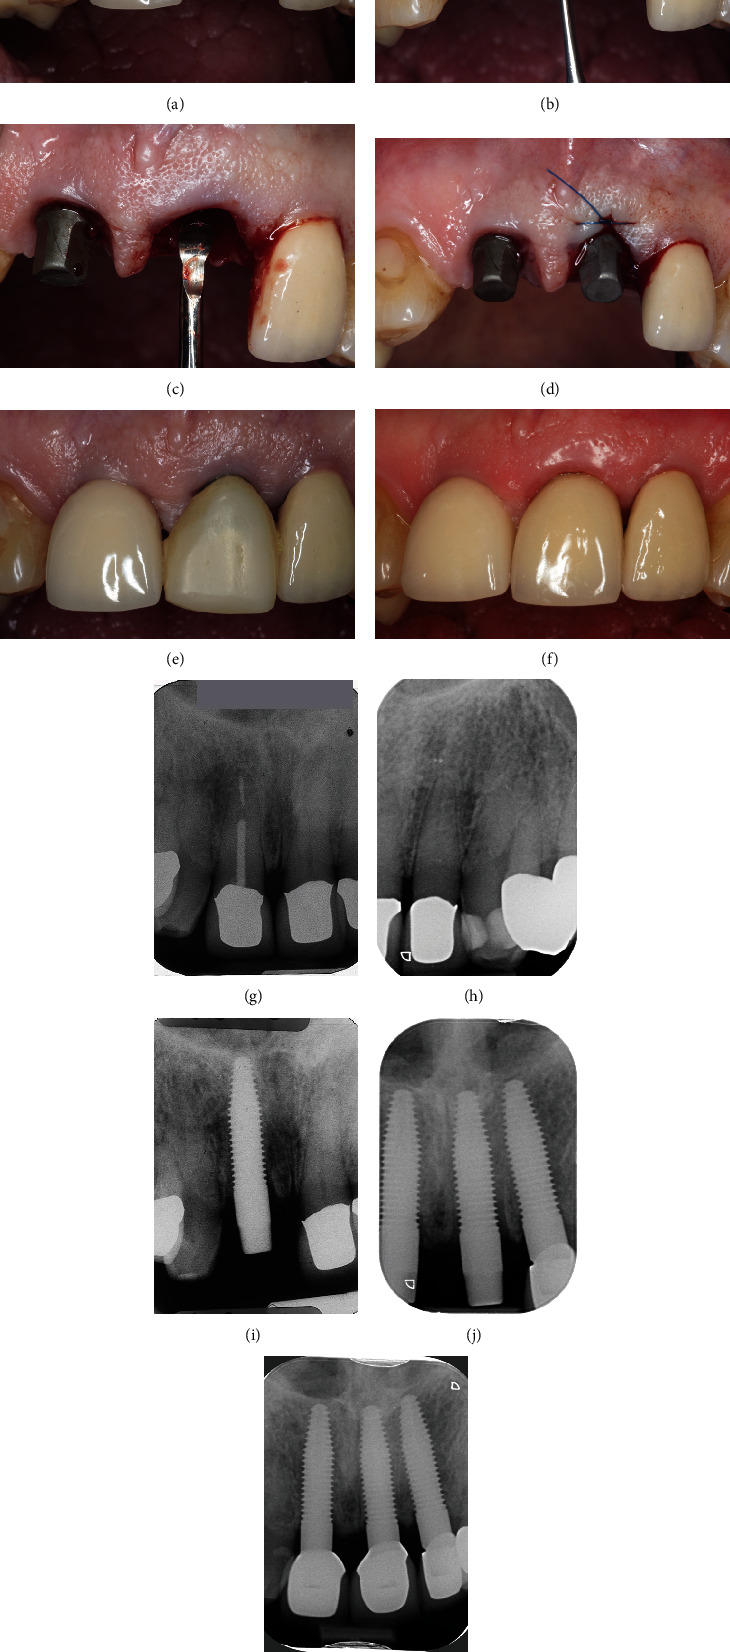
Immediate restoration of one-piece implant in esthetic zone: (a) clinical situation after fracture of left central incisor, (b) atraumatic extraction of remaining tooth, (c) complete extraction and cleaning of alveolar ridge, (d) clinical situation after immediate implant placement, (e) provisional restoration, reattachment of implant crown for right central incisor, (f) prosthetic restoration with single crown five months after healing, (g) initial radiographic situation with hopeless right central incisor, (h) radiographic situation before implantation of left lateral incisor, (i) radiographic control after implantation of first right incisor, (j) radiographic control after immediate implantation of left central incisor, and (k) radiographic situation nine months after immediate implant placement for left central incisor.
